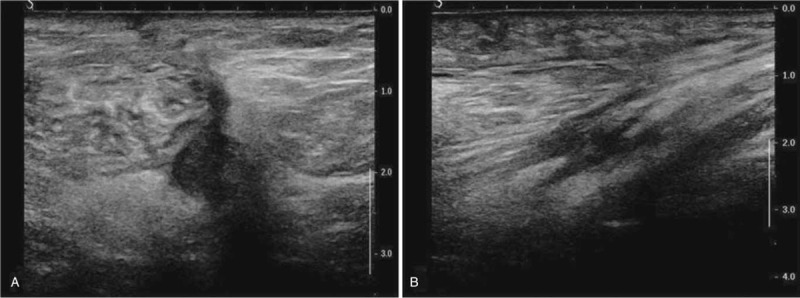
Two months after surgery, follow-up transverse (A) and longitudinal (B) ultrasonography images show no evidence of recurrent or residual lesions, and only show typical postoperative changes in the posterolateral aspect of the left knee.
